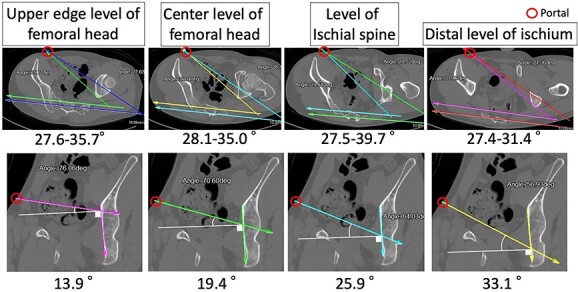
Estimated screw angles, Example CT images are shown from the upper edge level of the femoral head to the distal part of the ischium. The upper row shows inclined axial plane images, and the lower row shows inclined coronal plane images. Estimated screw angles to plate from the portal site to the posterior column are measured.
